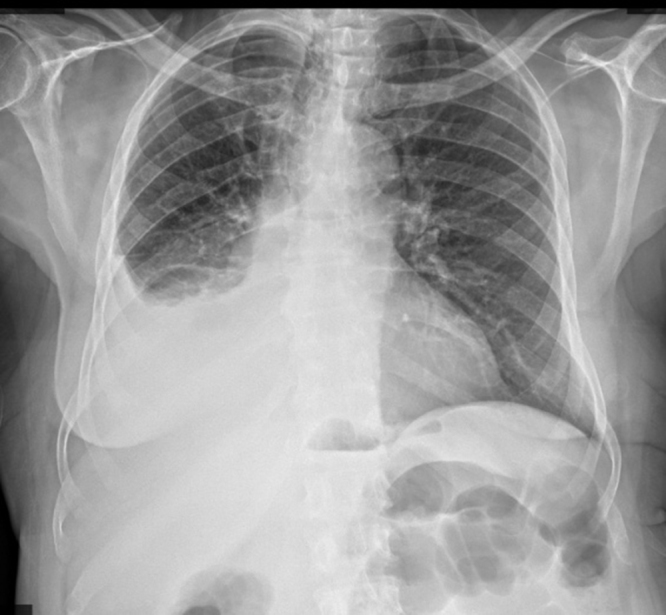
Chest X-ray in posterior aterior view. Homogenous opacity obliterating the right costophrenic angle with reduced right lung volume on the right hemi-thorax.
